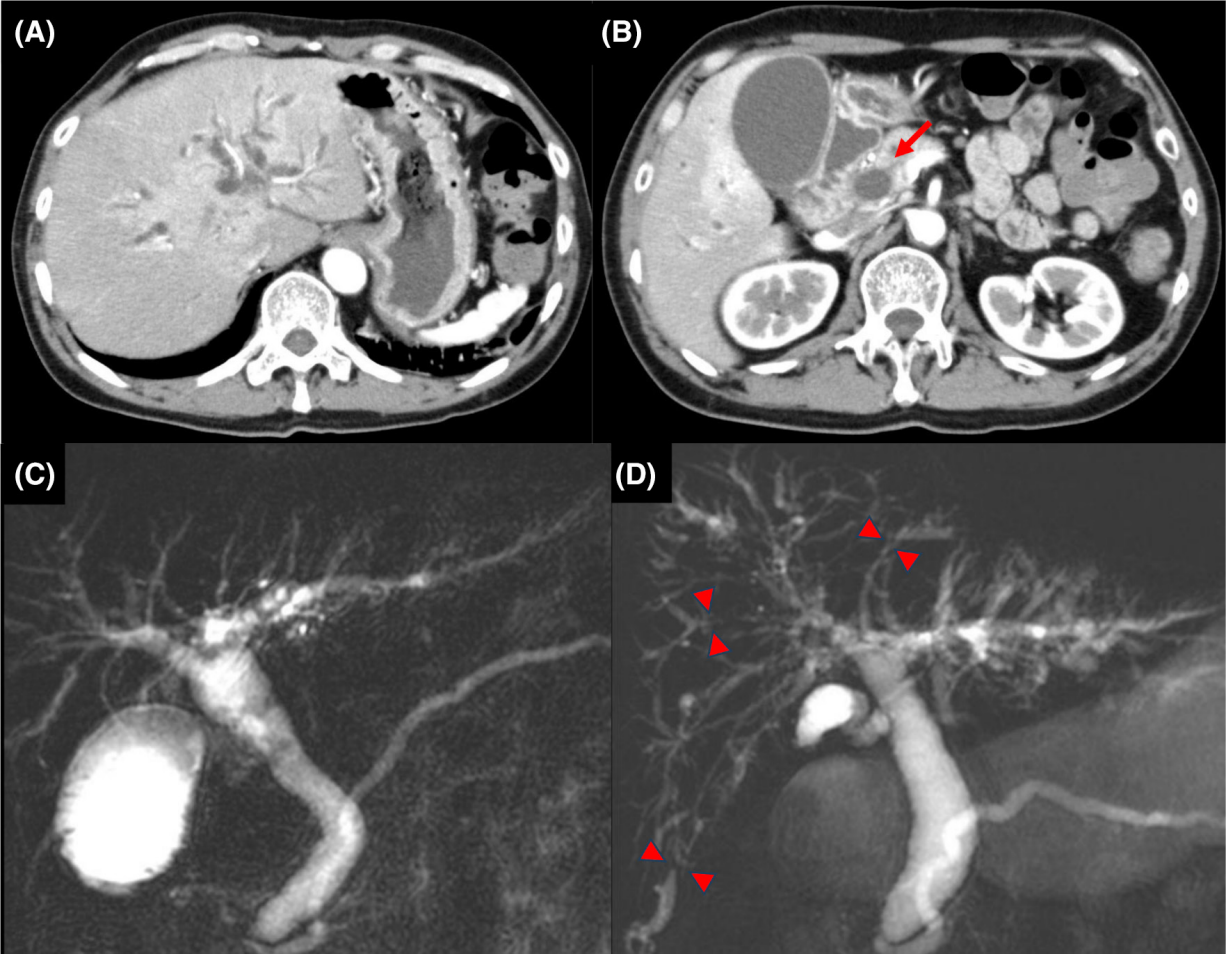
Abdominal contrast‐enhanced computed tomography (CECT) and magnetic resonance cholangiopancreatography (MRCP) findings. (A), (B) CECT image on day 58 after pembrolizumab shows intrahepatic bile duct dilatation, common bile duct dilation, and wall thickening (arrow). (C) MRCP on day 58 after pembrolizumab treatment shows common bile duct dilation. (D) MRCP on day 240 after pembrolizumab shows irregularly narrowed intrahepatic bile ducts (arrowheads) and dilation of the peripheral bile ducts.
